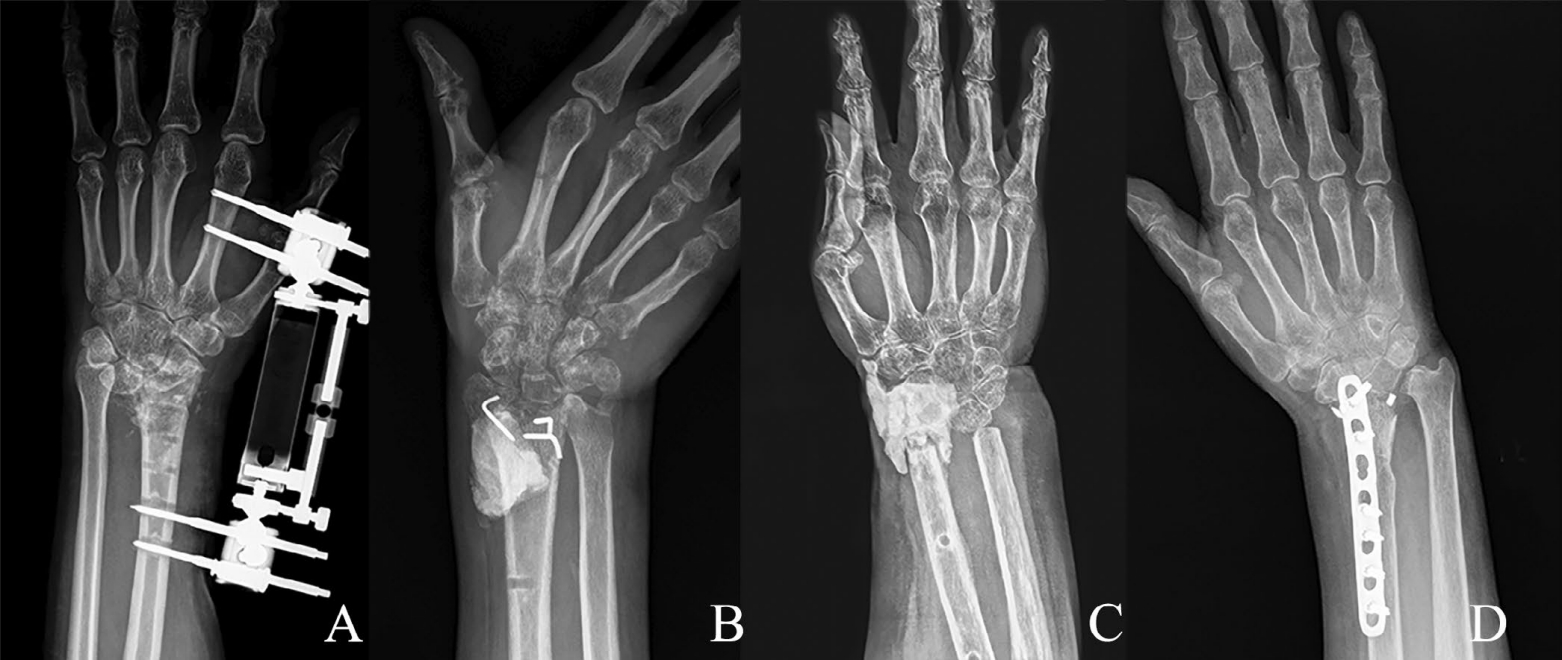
Preoperative radiographies of patients. (A) Patient 1: 3 months after plate removal and the external fixator lengthening procedure. (B) Patient 2: 1 month after the removal of the Kirschner wires and bone cement. Narrowing of the radiocarpal space, destruction of the articular surface, and osteoporosis of the carpal bones are observed. (C) Patient 3: 6 months after cement filling. (D) Patient 4: 3 years after the excision of a GCT and allograft reconstruction.
